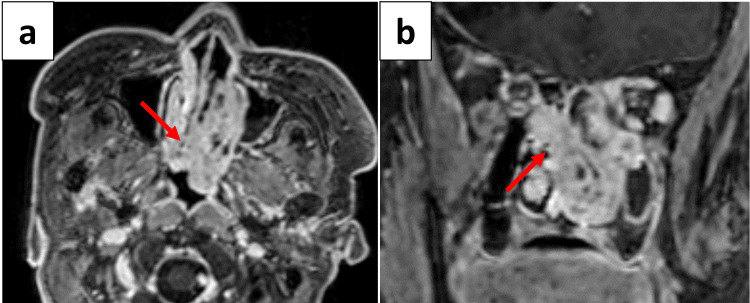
Preoperative sinonasal contrast MRI findings. Axial (a) and coronal (b) sections showing the mass signal with high contrast effect centered in the left nasal cavity. No obvious dural or orbital invasion was identified; however, the mass penetrated the nasal septum and extended into the right nasal cavity (arrows).
